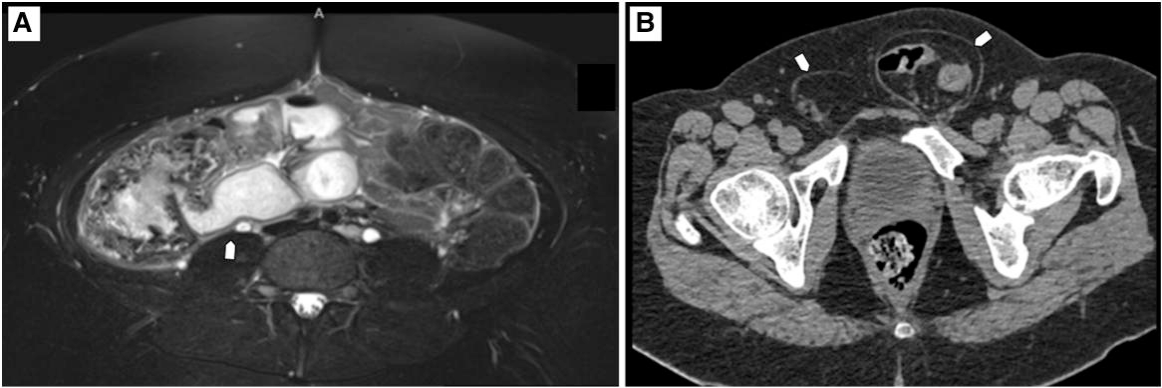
A, T2-weighted MRI scan showing an intra-abdominal mass and pyosalpinx in a 13-year-old girl with PWS. B, CT scan showing bilateral inguinal hernia in a 38-year-old male with PWS.
